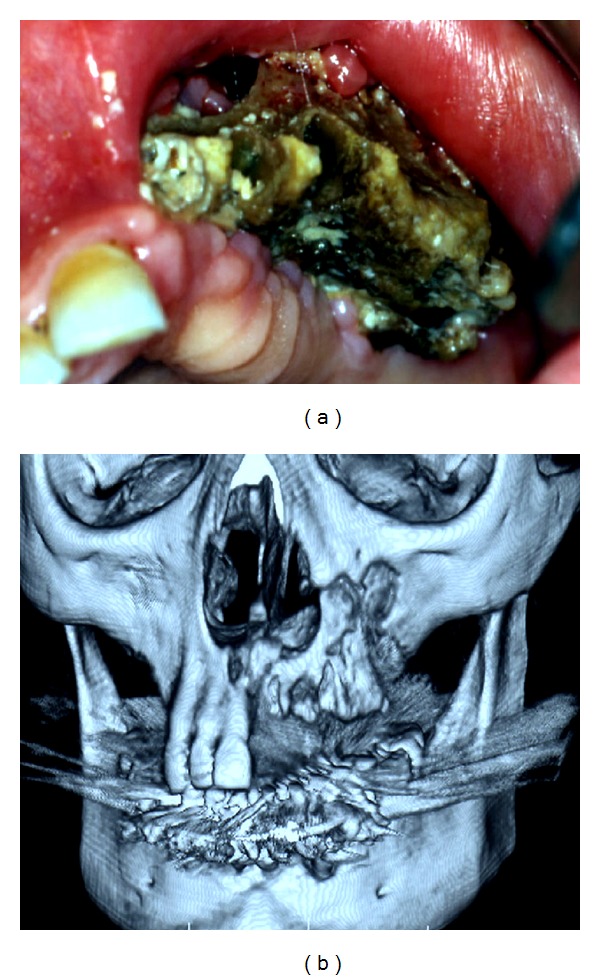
Clinical aspect and multislice spiral CT with 3D reconstruction of Stage III BRONJ involving the maxillary sinus, in a 74-year-old female patient with multiple myeloma, who underwent zoledronic acid therapy.
